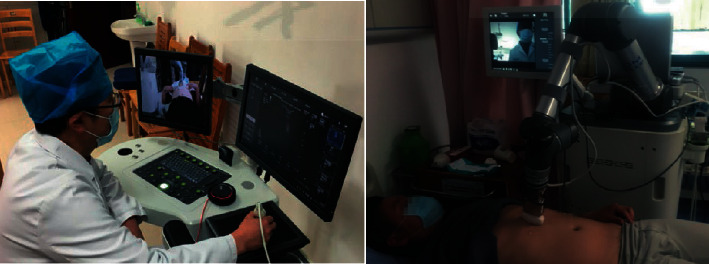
Remote ultrasonic inspection of 5G robots.
